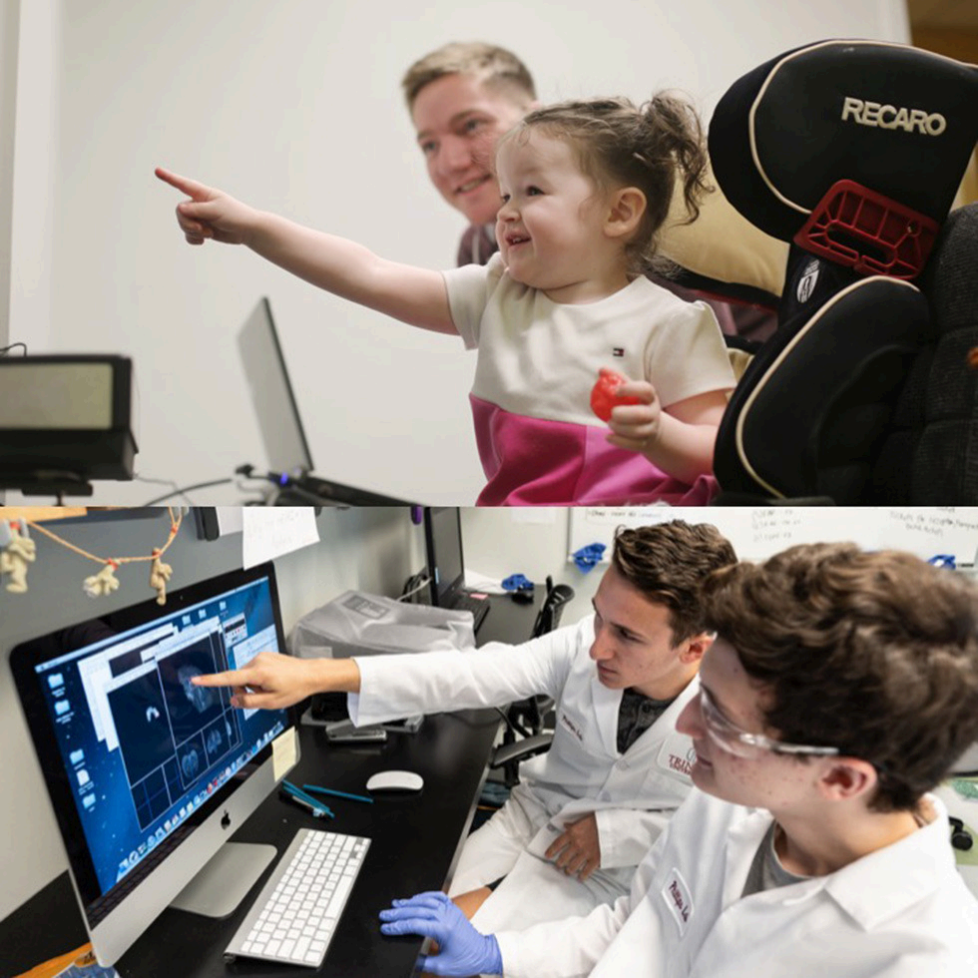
Undergraduate Research assistant collecting data from a child using an eye-tracker (top panel); undergraduates discussing analysis of MRI scan (bottom panel). Written informed consent was obtained from the depicted adults and students, and the parents of the depicted child, for the publication of this image. All appropriate permissions were obtained from the University/copyright holders for the use of this image in the manuscript. Photo credit: Trinity University.
